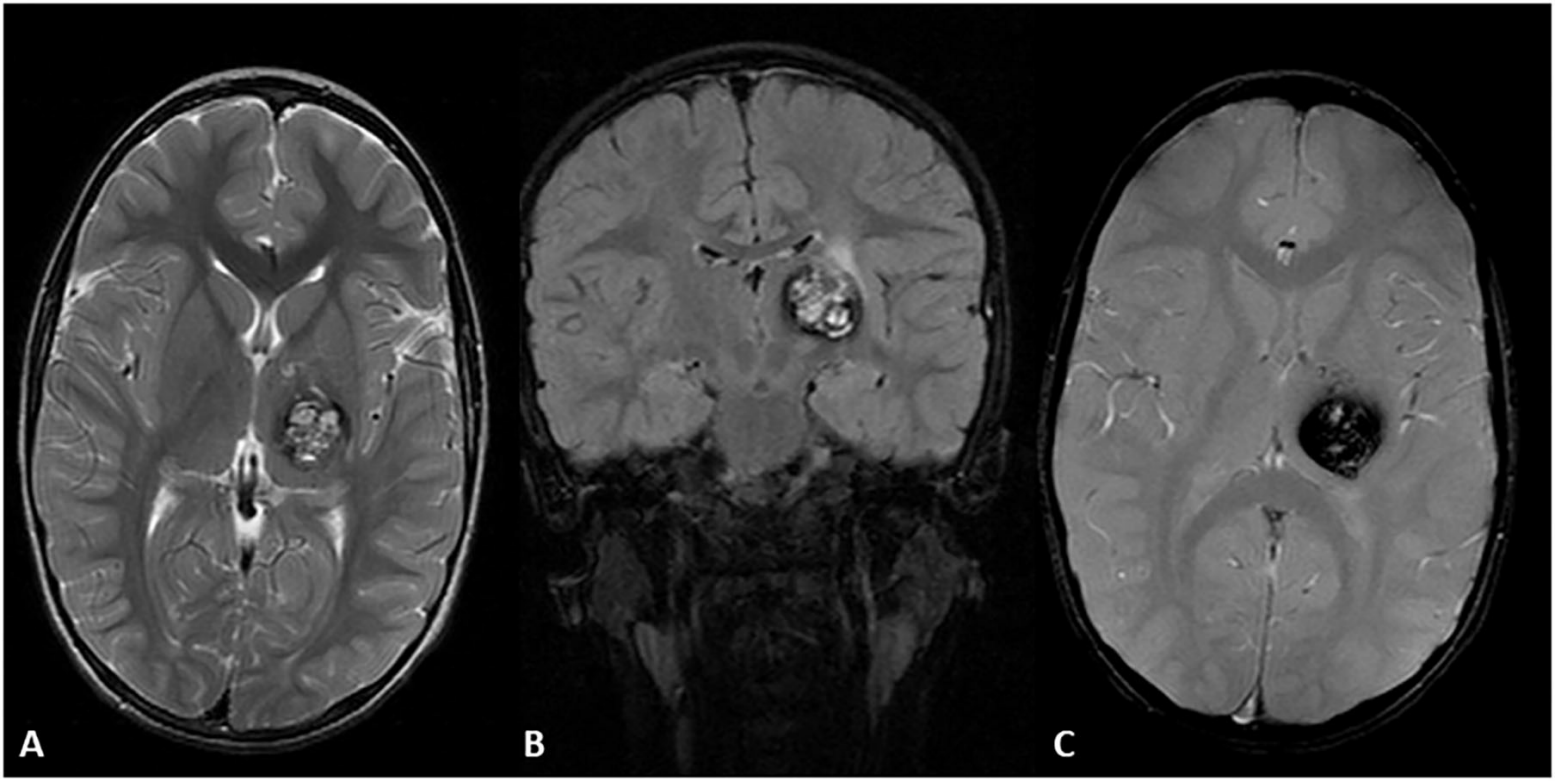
Four-year-old Hispanic girl presented with right sided tremor in the context of a new lesion compatible with cavernous malformation with subacute bleeding of the left thalamus. MRI axial T2 sequence (A) heterogenous hyperintense lesion with a hypointense rim due to subacute internal bleeding know as a “popcorn” appearance. MRI coronal FLAIR (B,C) demonstrates the same lesion with surrounding edema in the upper region of the lesion. MRI axial GRE T2 sequence demonstrates a prominent blooming artifact. T1 post-contrast showed no enhancement (not shown).
